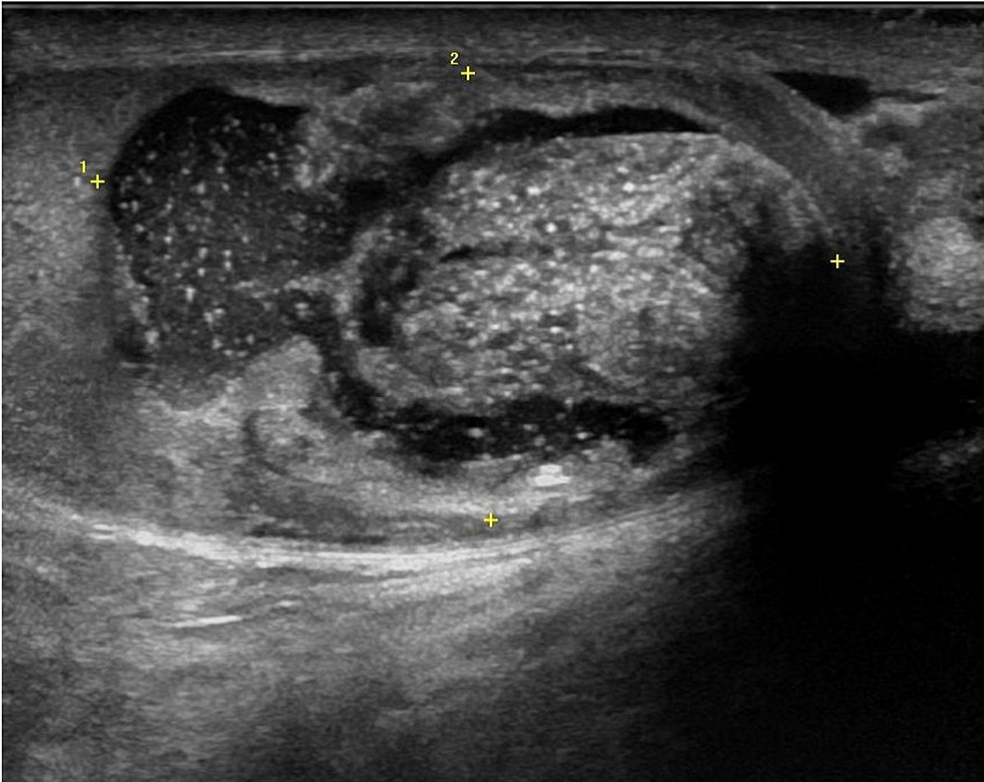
Ultrasound of the testes showing a left testicular mass (encompassed within the yellow plus symbols).
